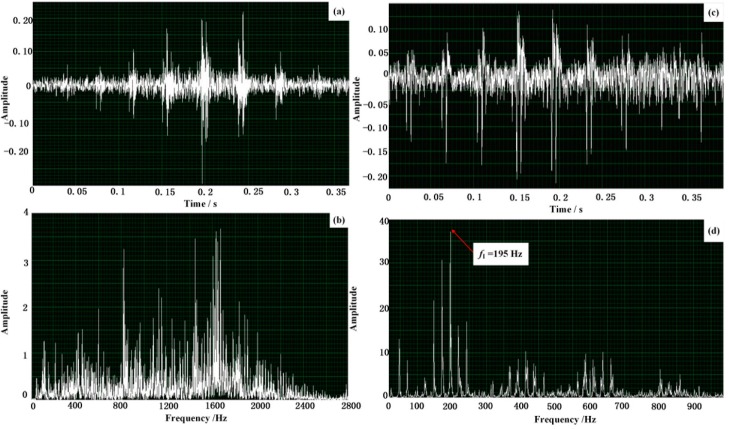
Effectiveness verification via the designed embedded system: (a,b) the waveform and the spectrum of the input Doppler distorted signal of the defective train bearing with a defect on the inner-race; (c,d) the waveform and the spectrum of the output restored envelope signal via the designed embedded system.
